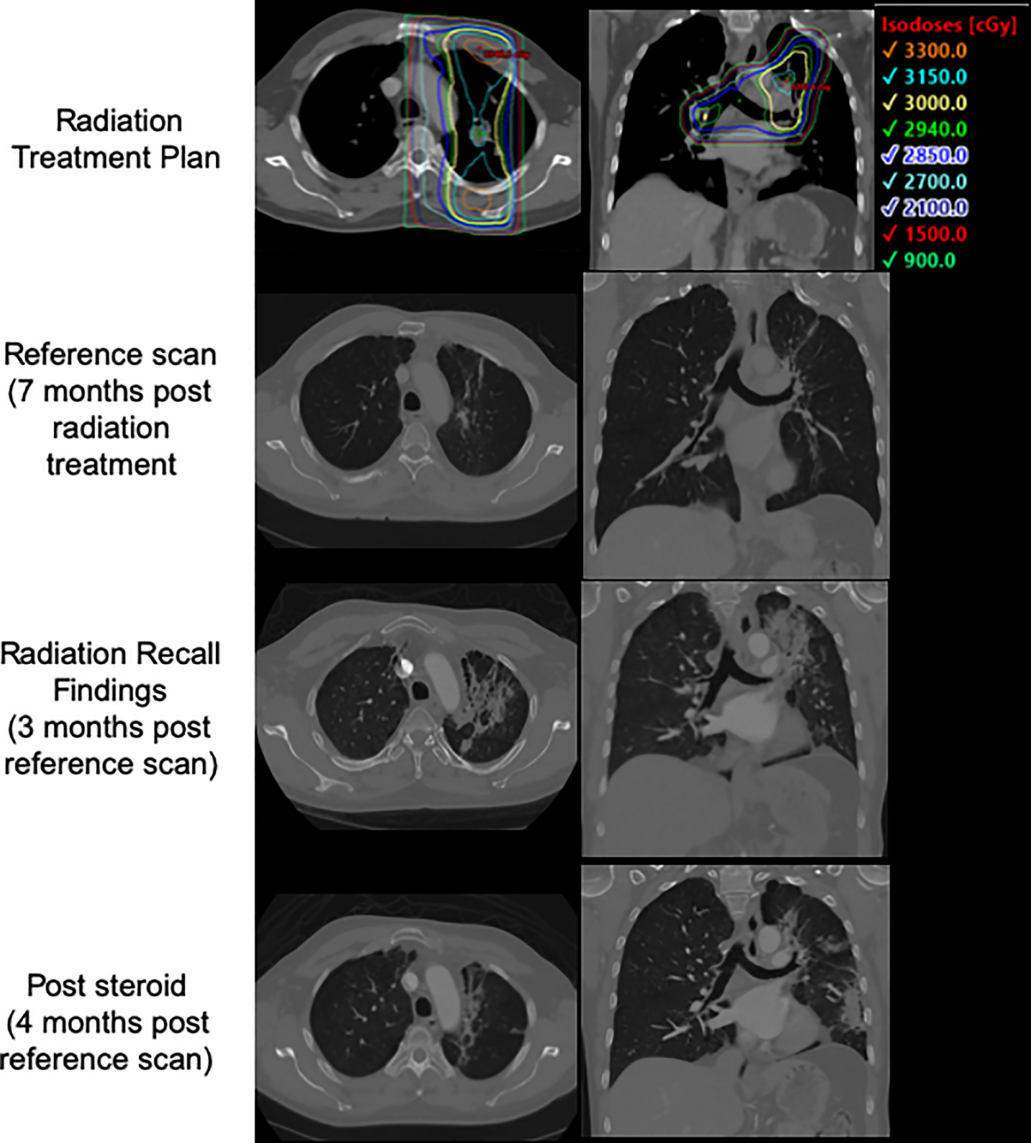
Patient 3's treatment plan, post treatment CT, CT at time of presentation with radiation recall, and CT following course of steroids with interval improvement.
